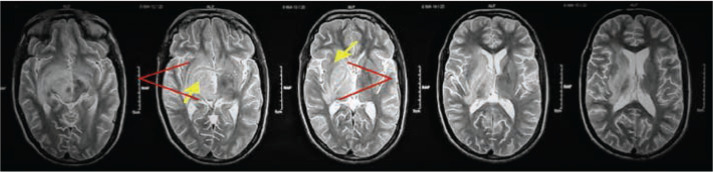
Axial fluid-attenuated inversion recovery MRI showing extensive hyperintensity with mild mass effect involving the right temporal lobe, basal ganglia, internal capsule, and thalamus suggesting the classic/cascade sign of NBD (red bracket showed extension while yellow arrow indicates the hyperintense lesion.
